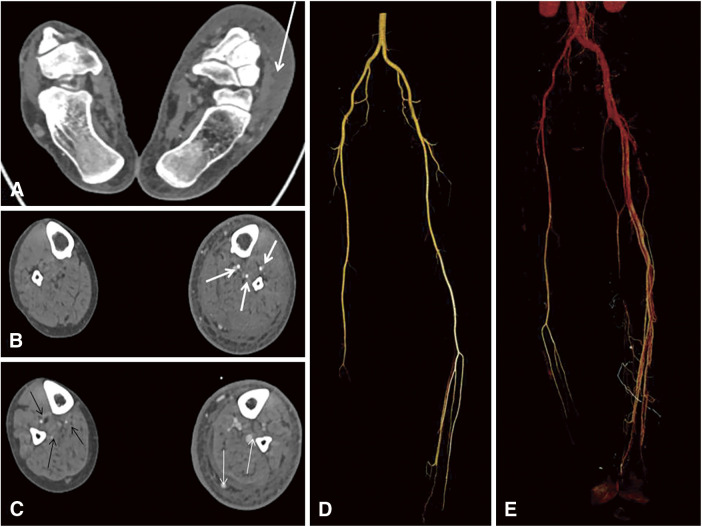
Fig. 2 (A) Axial CT image of the lower limbs at the level of the ankle shows diffuse subcutaneous edema involving the left lower leg and foot (white arrow). (B) Arterial phase CT angiography image shows luminal enhancement of the left anterior tibial, posterior tibial, and peroneal arteries (white arrows). There is no corresponding luminal enhancement of the right lower limb arteries. (C) The venous phase image shows good venous filling in the left lower limb (white arrows). On the right side, considerable lag is noted, with mild luminal enhancement of the right anterior tibial, posterior tibial, and peroneal arteries (black arrows). (D) 3D reconstruction of CT angiography run in the arterial phase shows a considerable lag of luminal enhancement of right lower limb arteries as compared to the left side. (E) 3D reconstruction of the venous phase run shows good venous return from the left lower limb while further distal luminal enhancement in the right lower limb arteries is noted and still lagging the left lower limb. CT: computed tomography
